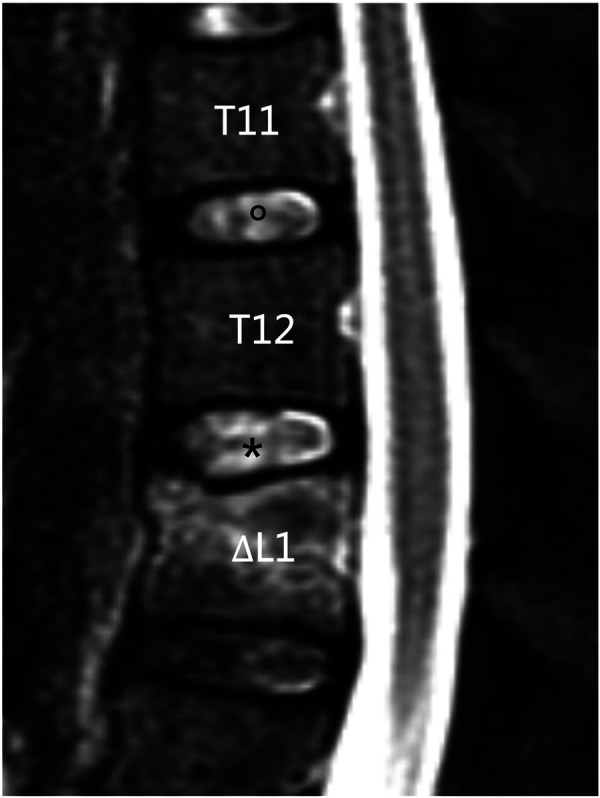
Definition of adjacent discs and control discs. In the mid-sagittal view of MRI, L1 was the fractured vertebral body, T12/L1 (*) disc was defined as the adjacent disc, and T11/T12 (∘) disc was defined as the control disc.
